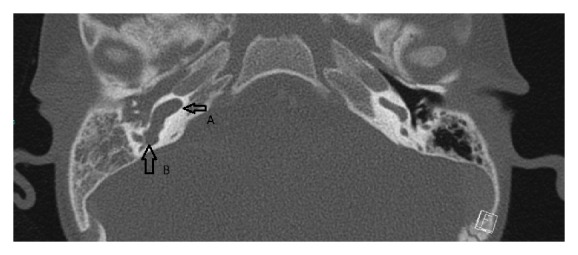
Computed tomography of the temporal bone showing the cystic malformation in the cochlea (A) and the vestibular dilatation (B).
